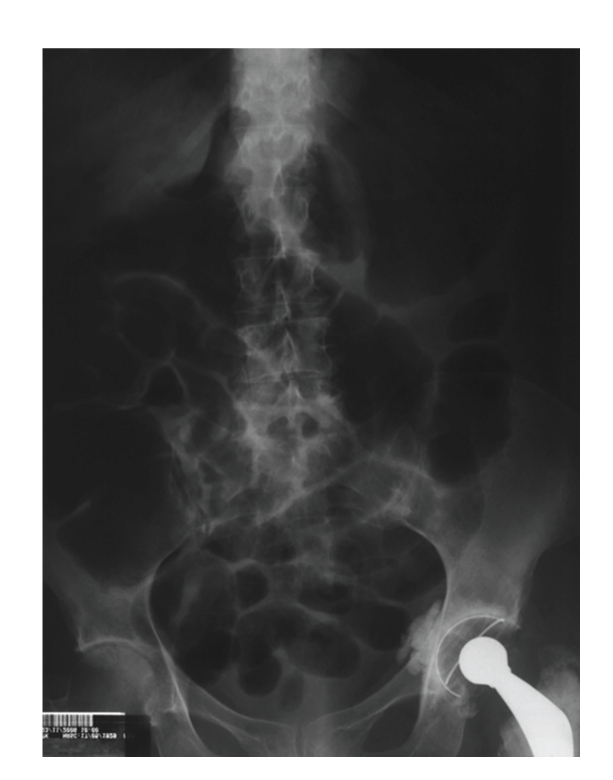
Abdomen radiograph showing the presence of high level of air fluid in the bowel loops.
